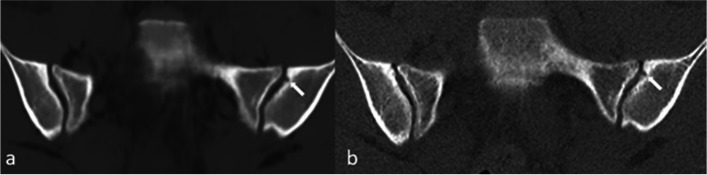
Joint facet defect ≥ 3 mm (arrows) in the left iliac joint in a 16-year-old girl in a paracoronal plane. a MRI-based synthetic CT (sCT). b CT
